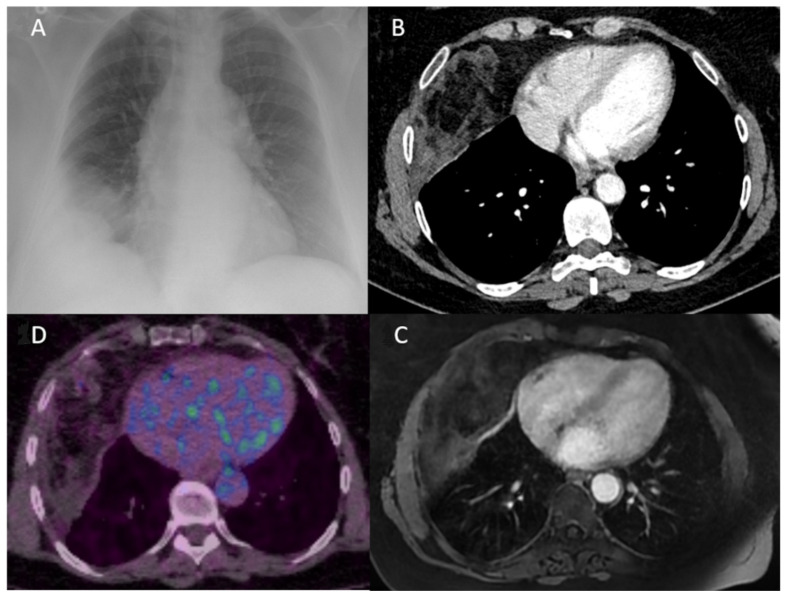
(A) Chest X-ray showing a non-systematized opacity of the right costo-diaphragmatic angle making the diagnosis between pleural effusion and thoracic mass difficult. (B) CT scan of the chest (axial view) confirming the presence of a heterogeneous intra thoracic mass not appearing to be in the lung parenchyma. (C) An axial T1-weighted MR image of the chest identifying a tumorous chest wall mass with both fat and tissue components, suggestive of liposarcoma. (D) 18-fluorodeoxyglucose (FDG) positron emission tomography showing a low hypermetabolism of this lesion with a standardized uptake value of 1.8.
